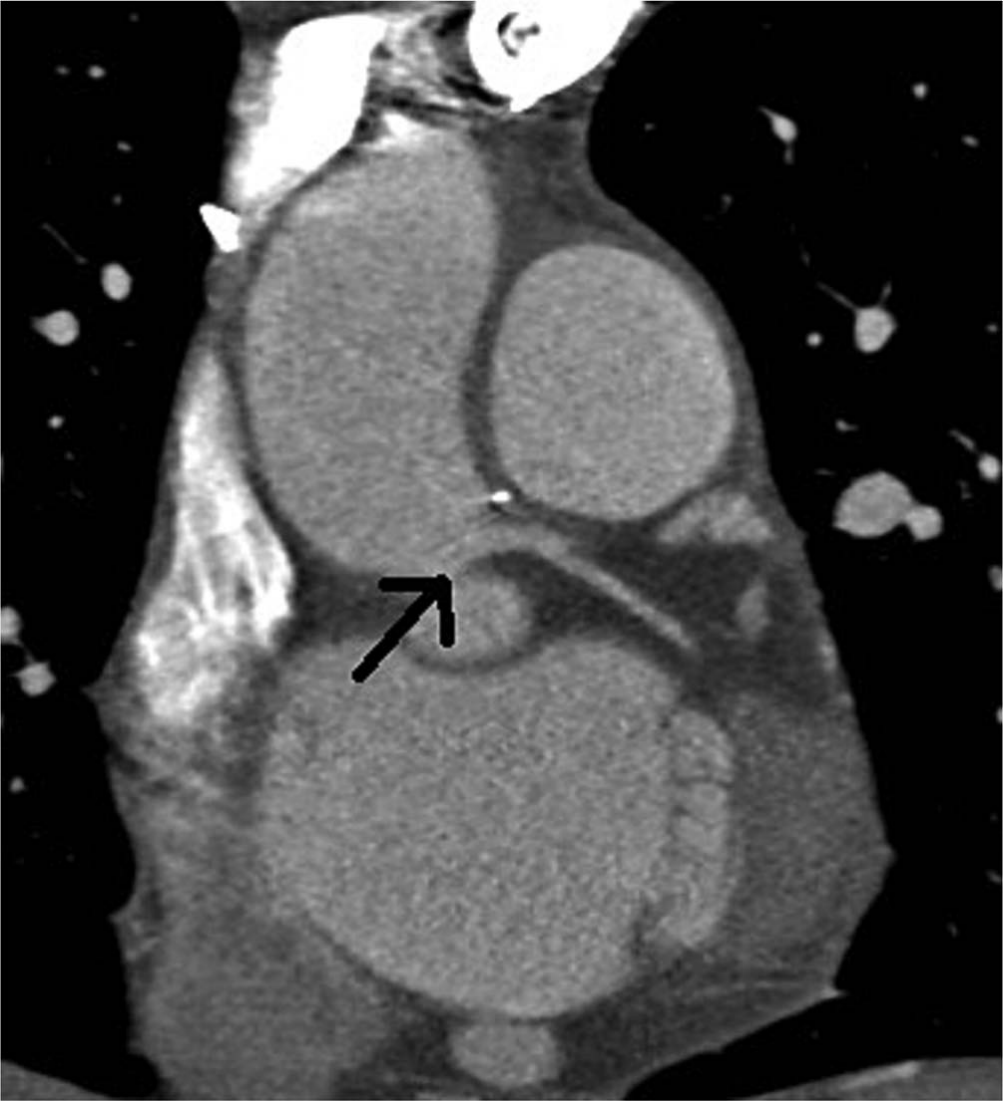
Postoperative multislice computed tomographic coronary angiography showing no signs of recurrent stenosis, with a widely open left main coronary artery (arrow)
